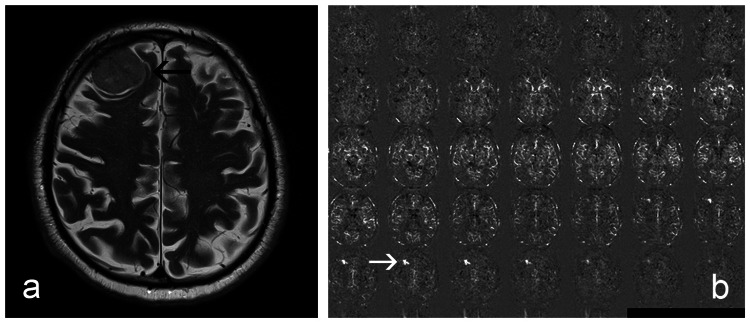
Meningioma adjacent to the frontal bone on the right. (a) T2-weighted image with the black arrow indicating tumour. (b) ASL images show increased signals in some areas (white arrow).
